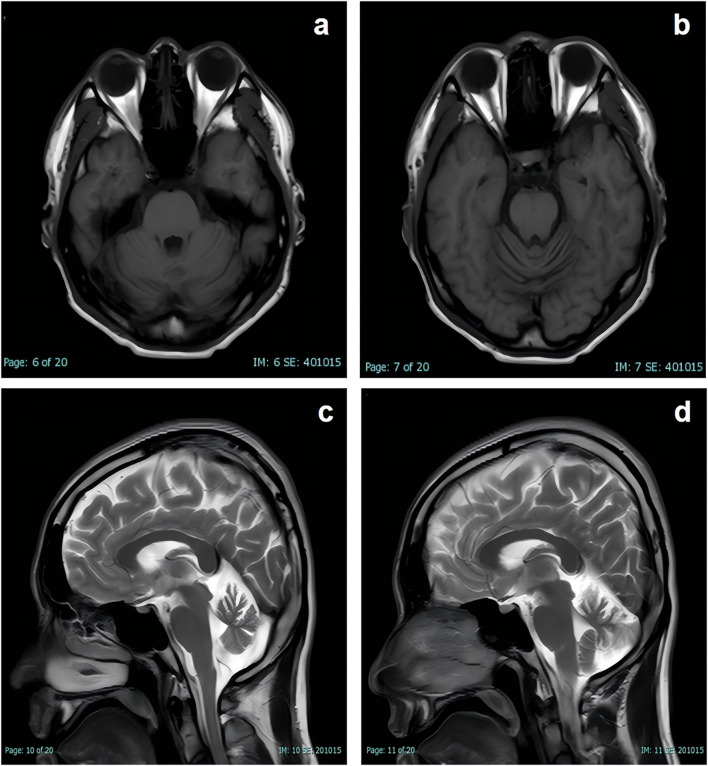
Brain MRI shows marked cerebellar atrophy. (a,b) Axial T1-weighted images demonstrate reduced cerebellar volume; (c,d) sagittal T2-weighted images further delineate cerebellar folia thinning.
